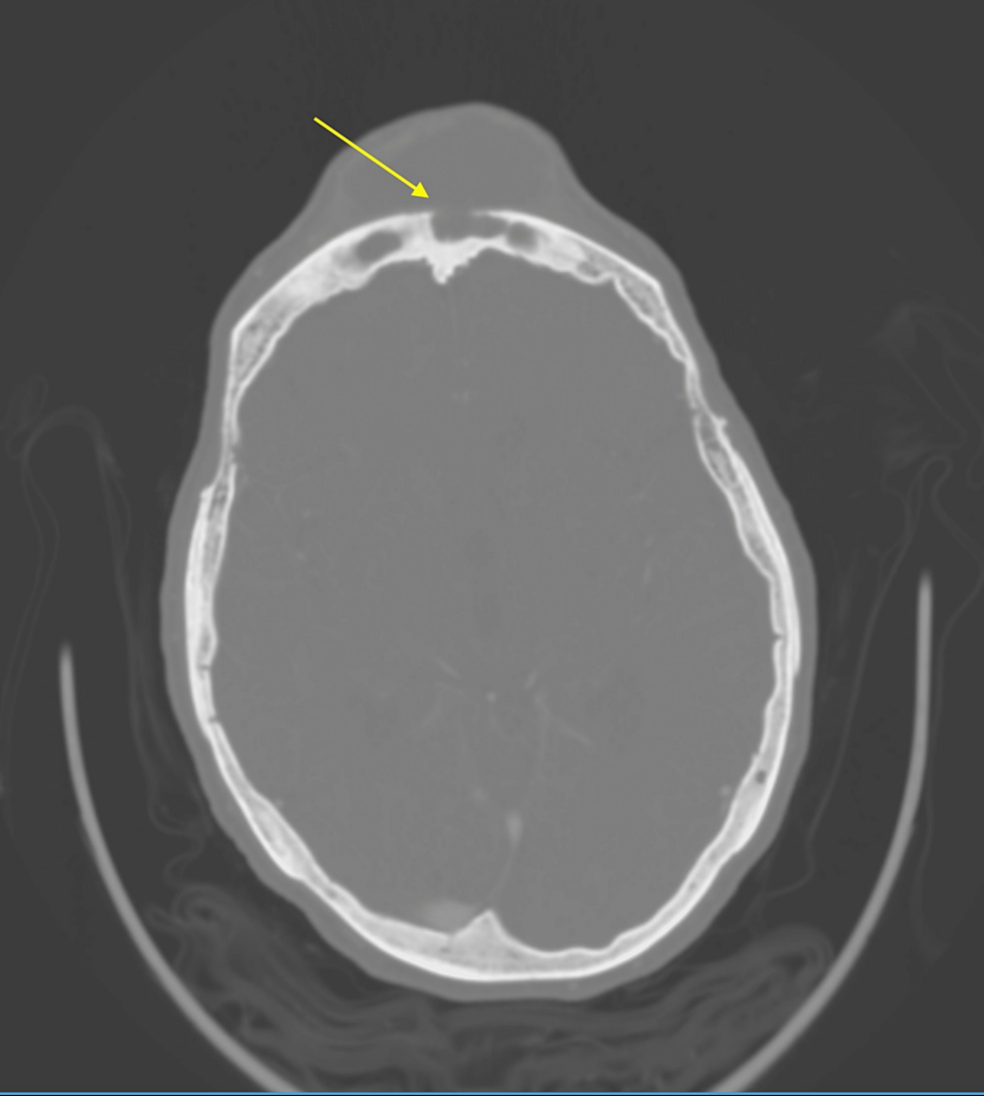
CT scan of the head showing a large, likely chronic subcutaneous collection in the midline frontal region with the erosion of adjacent frontal bone (yellow arrows) with possible communication to the left frontal sinus.
